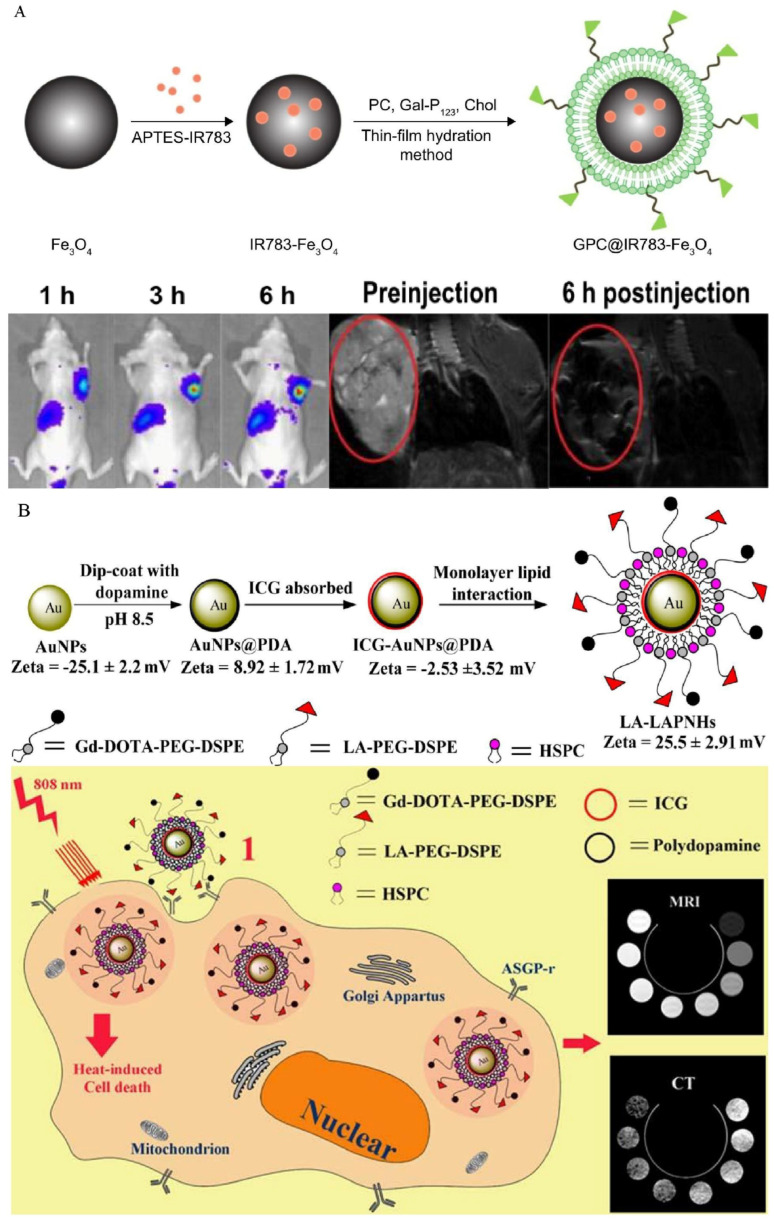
(A) The synthesis of MRI/NIRF dual-modal probe and effect of imaging. Reproduced with permission [82]. Copyright 2018, Dovepress. (B) The composition of MRI/CT dual-modal probe and theranostic mechanism. Reproduced with permission [83]. Copyright 2014, American Chemical Society.
